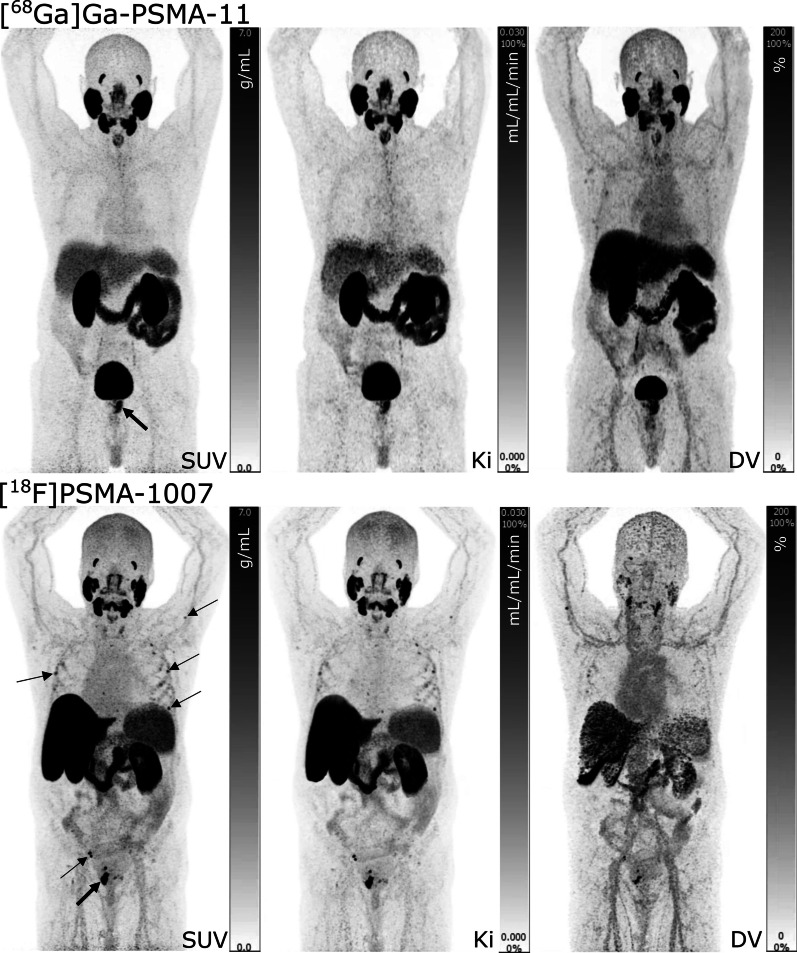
Examples of D-WB PET/CT scans performed in patients referred for primary staging of prostate cancer. SUV images are reconstructed using D-WB data from 60 to 70 min, whereas the parametric images of Ki and DV are reconstructed using D-WB data from 40 to 70 min. Above: the example of a D-WB PET/CT scan performed with [^68^Ga]Ga-PSMA-11 displaying only primary disease (arrow). Below: one of the patients scanned with [^18^F]PSMA-1007 showing primary disease in the prostate (thick arrow), dissemination to pelvic lymph nodes, as well as multiple small skeletal lesions in the ribs and left humerus (probably unspecific/benign) (thin arrows)
